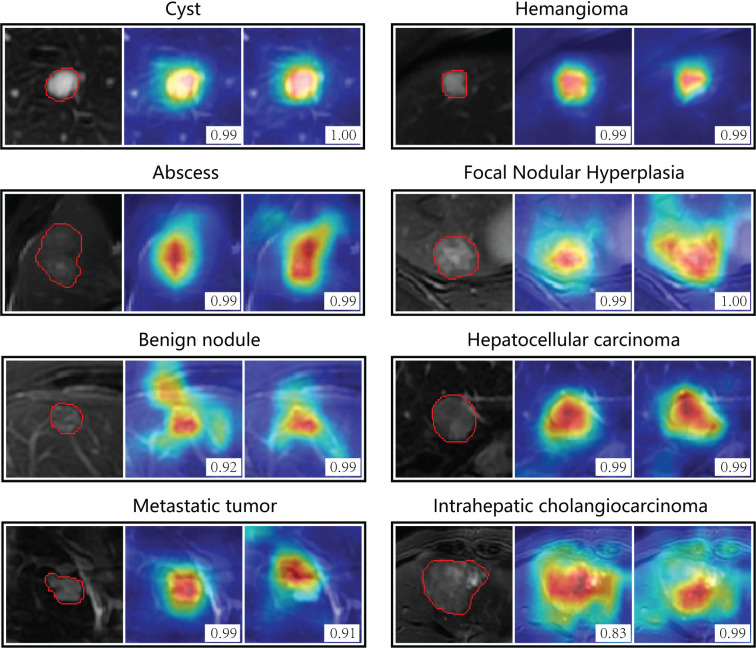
Attention maps of non-contrast models on eight types of focal liver lesions. The color-coded maps highlight regions which were most discriminative for a certain category. Red indicates the areas that contributed most, and blue areas contributed least. The left column is the region of interest from T2 image, the middle is the attention map of T2 model, and the right is attention map of T2+DWI model. The number in the picture indicates the probability of corresponding category predicted by the model. The original T2 images for each lesion were presented in Supplementary Figure S4.
